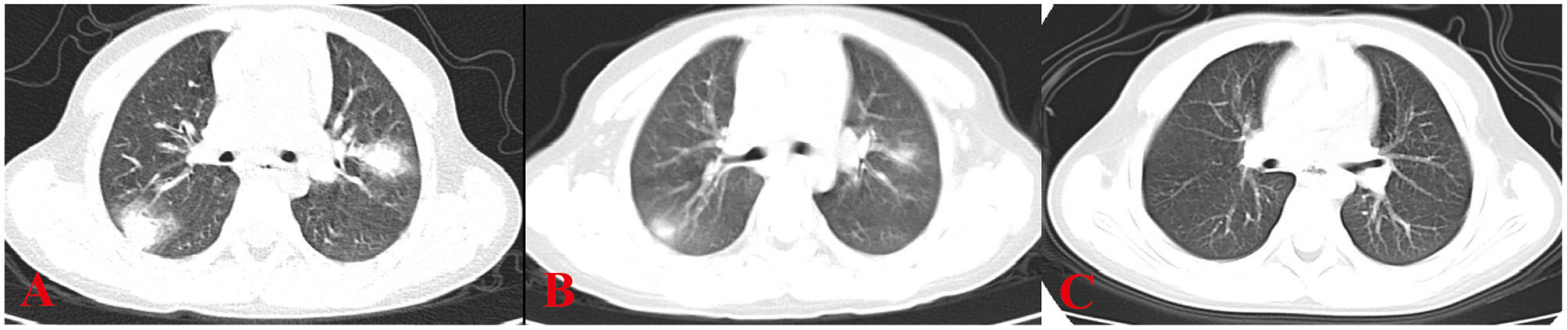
HRCT sign changes of case 1. (A) Multiple masses of ground glass–like increased density can be seen close to the subpleural of the outer lung field, the center density was much higher than the periphery, and the periphery showed a halo sign or faint cloudiness. (B) After 1 week of treatment, the high-density shadows of both lungs are absorbed than before. (C) After 2 weeks of treatment, the peripheral lesions are absorbed before the central lesions and are finally completely absorbed without leaving any traces of the lesions.
